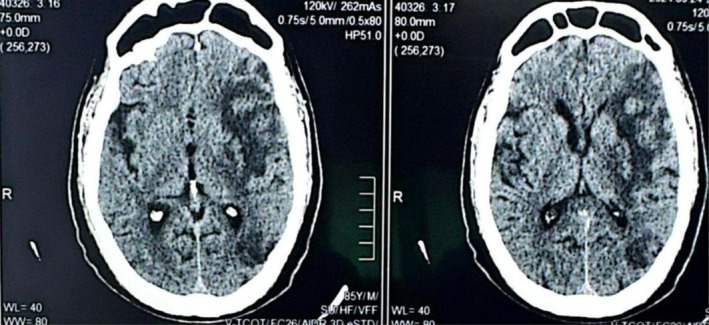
CT brain showing hypodense area in left temporal lobe, indicating an embolic infarct in the left middle cerebral artery (MCA).
